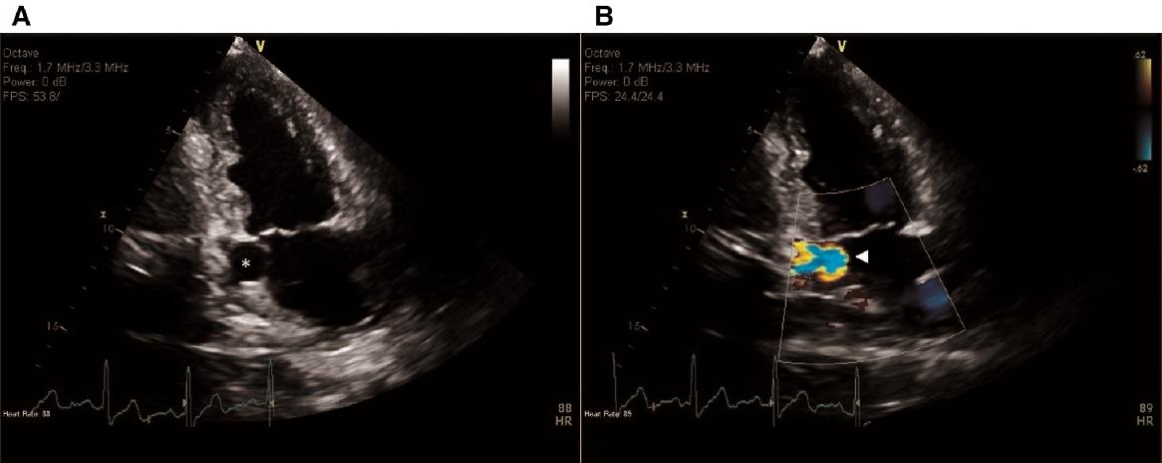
Initial transthoracic echocardiography. (*A*) Two-dimensional apical two-chamber view, showing a dilated coronary sinus (asterisk). (*B*) Colour Doppler imaging showed aliasing of colour in the coronary sinus, indicative of high velocity flow (arrowhead).
